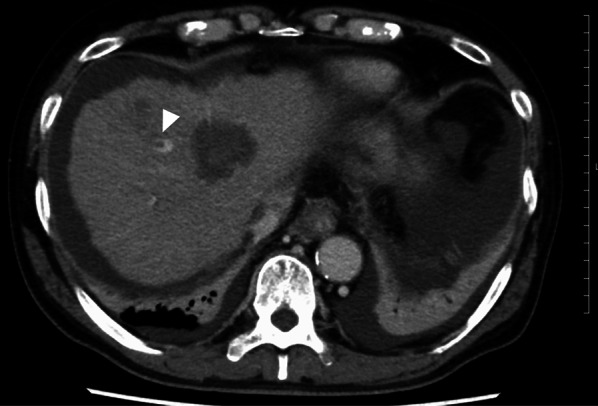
Abdominal computed tomography (CT) images of case 2. An enhanced abdominal CT scan revealed thrombosis in the branches of the portal vein (white triangle) on POD 5
